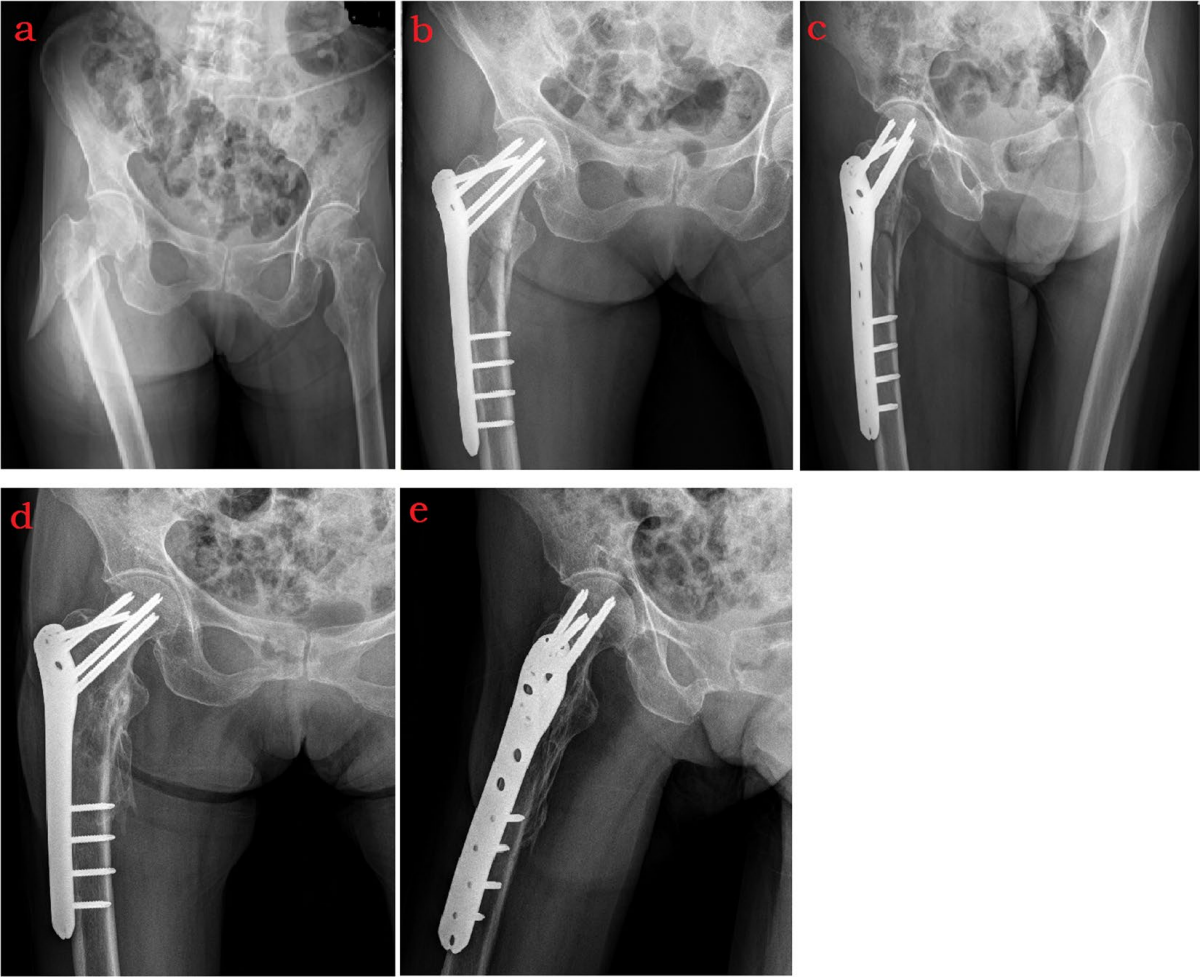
A 81-year-old female with right intertrochanteric fracture treated by proximal femoral locking plate (PFLP). (a) Radiograph of pelvis showed fracture of right intertrochanteric, with broken lateral wall. (b) Anteroposterior plain film of right hip taken 3 days after surgery showed satisfactory reduction of fracture. (c) Oblique plain film of right hip taken 3 days after surgery showed well aligned fracture, with stable internal fixation. (d,e) Fracture healing was showed in anteroposterior and oblique plain film of right hip taken 8 months after surgery.
